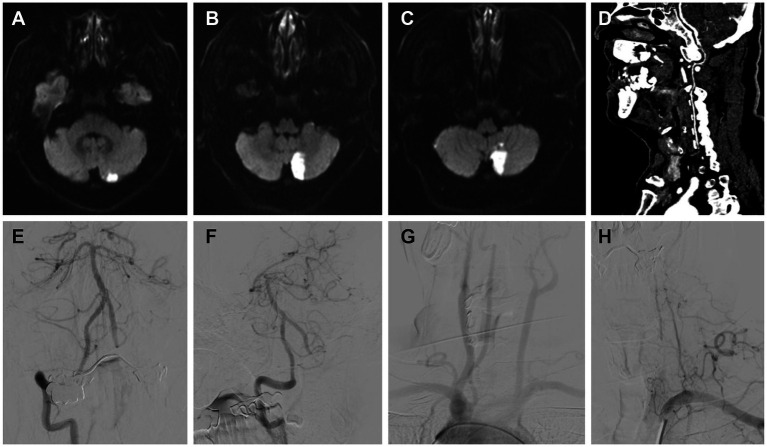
Imaging findings of Case 3. (A–C) DWI showing a new infarct in the left cerebellar hemisphere. (D) CTA showing occlusion of the left V1 segment. (E–H) DSA showing left V1 occlusion, with collateral flow from the ascending cervical artery and thyrocervical trunk and local turbulence.
